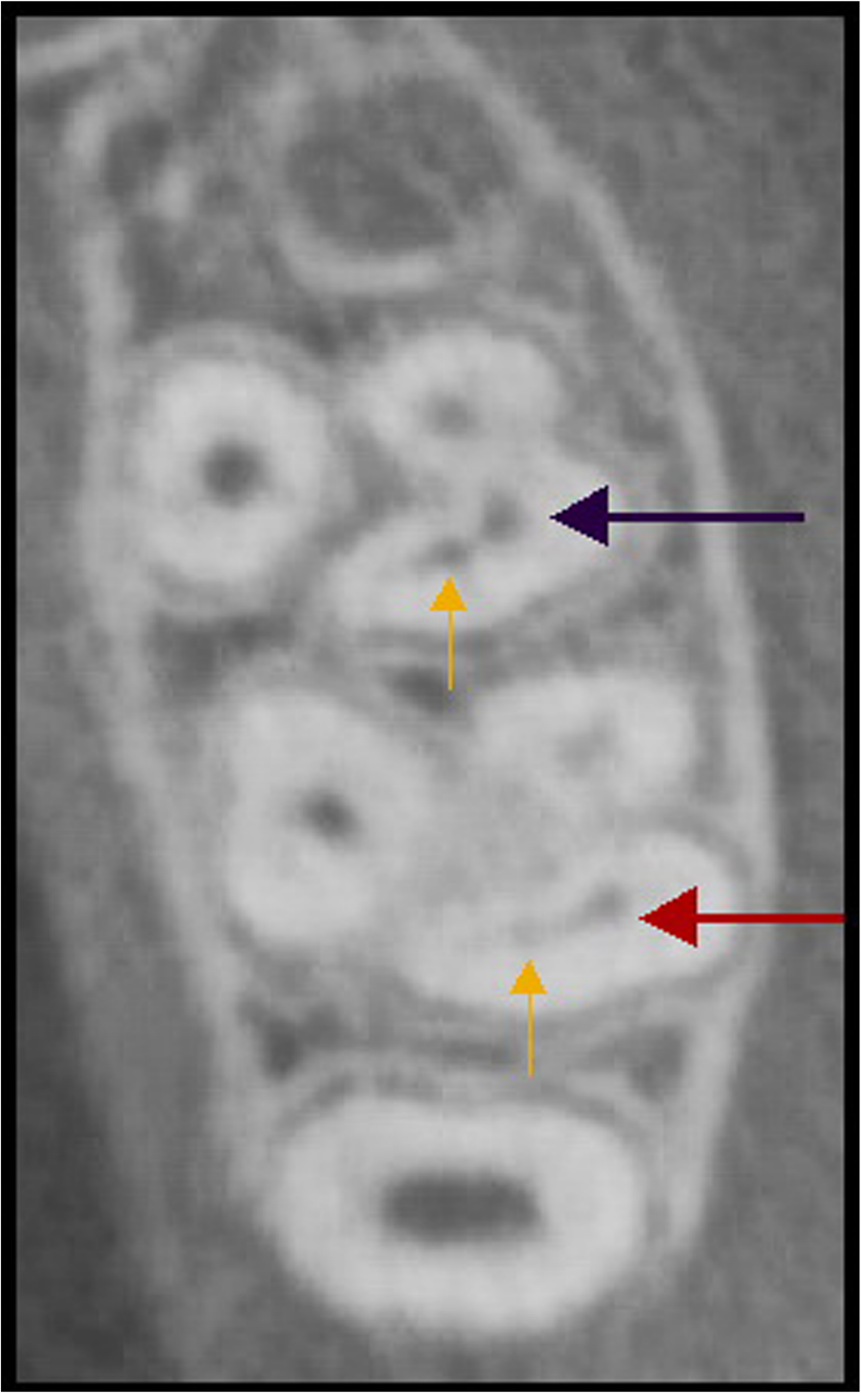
Cross-sectional CBCT image of left maxillary first and second molar with a clearly distinguished MB2 canal (yellow arrows). The red arrows denote the mesiobuccal root in the maxillary first molar and the purple arrows denote the mesiobuccal root in the maxillary second molar
